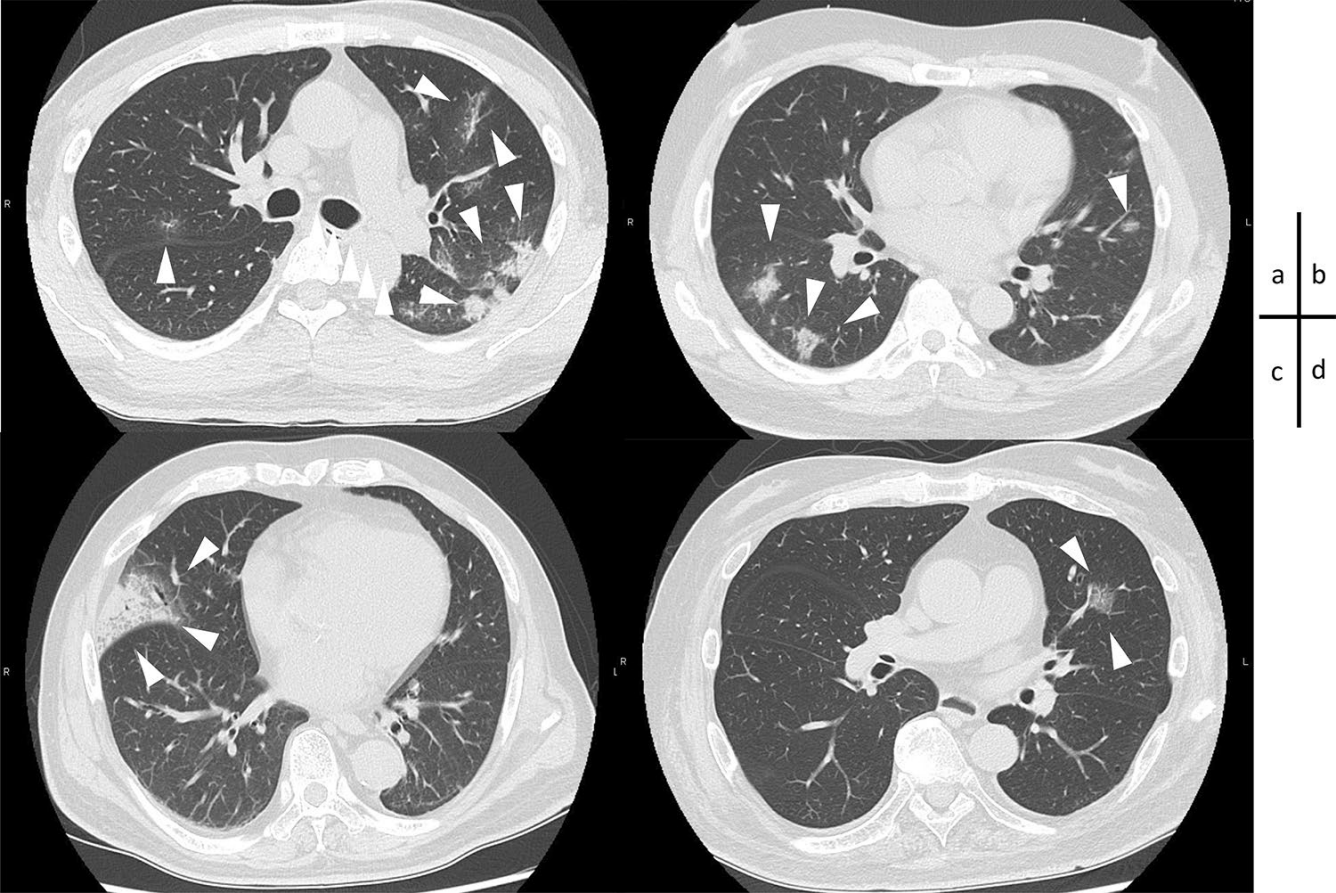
Chest HRCT images of patients treated with REGN-COV2 on admission (Case 1 to Case 4). Arrows indicate GGO considered to be caused by COVID-19. a Case 1; GGO bilaterally and infiltrative shadow with air bronchogram were shown in the left lower lung. b Case 2; multiple nodular to patchy opacities were revealed with peripheral predominance. c Case 3; GGO in the right middle lung. d Case 4; partial opacities located in the left lung. d Case 4; partial GGO in the left lung. *HRCT* high-resolution computed tomography, *GGO* ground
